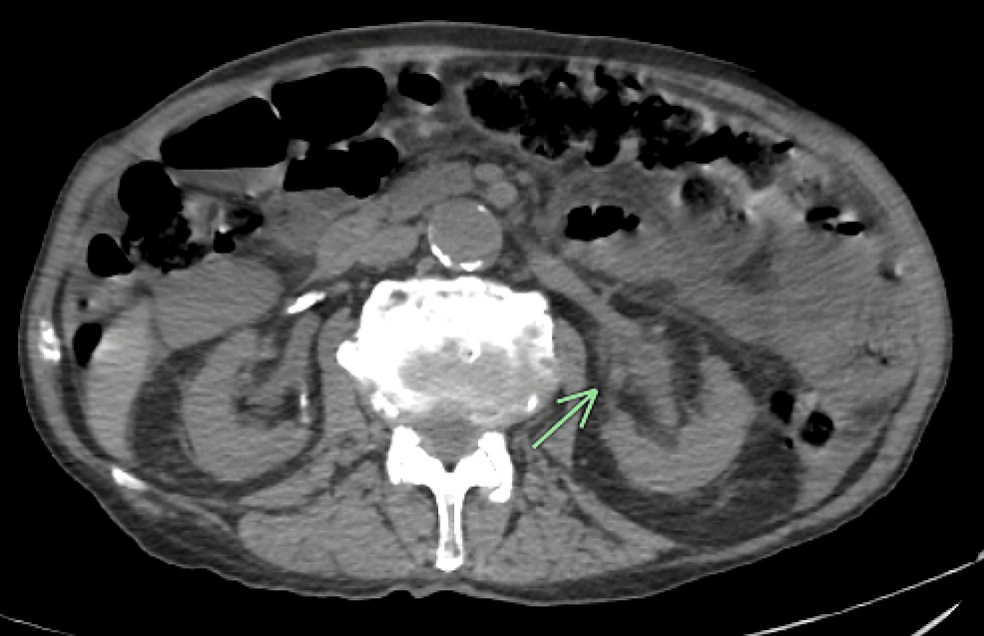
Abdominal and pelvic CT scan showing left hydronephrosis and hydroureter with the thickening of the renal pelvic mucosa CT: computed tomography
